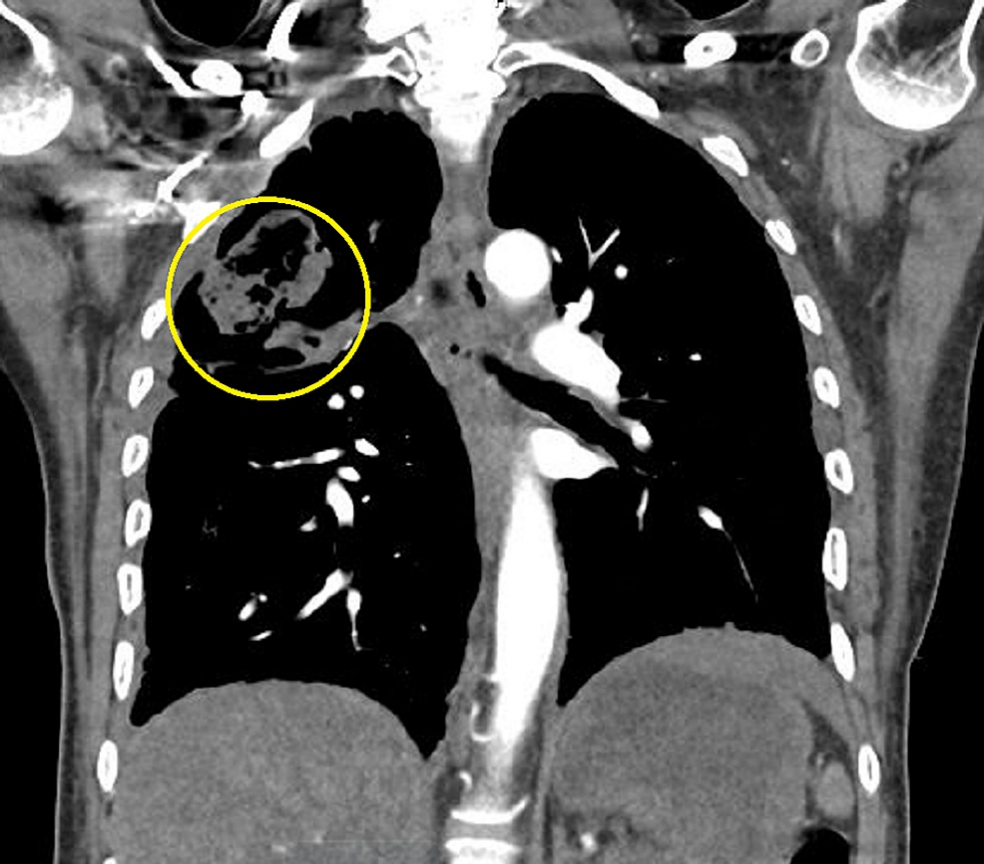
Coronal section of CT scan of the chest showing cavitary lesion in the right upper lobe of chest
